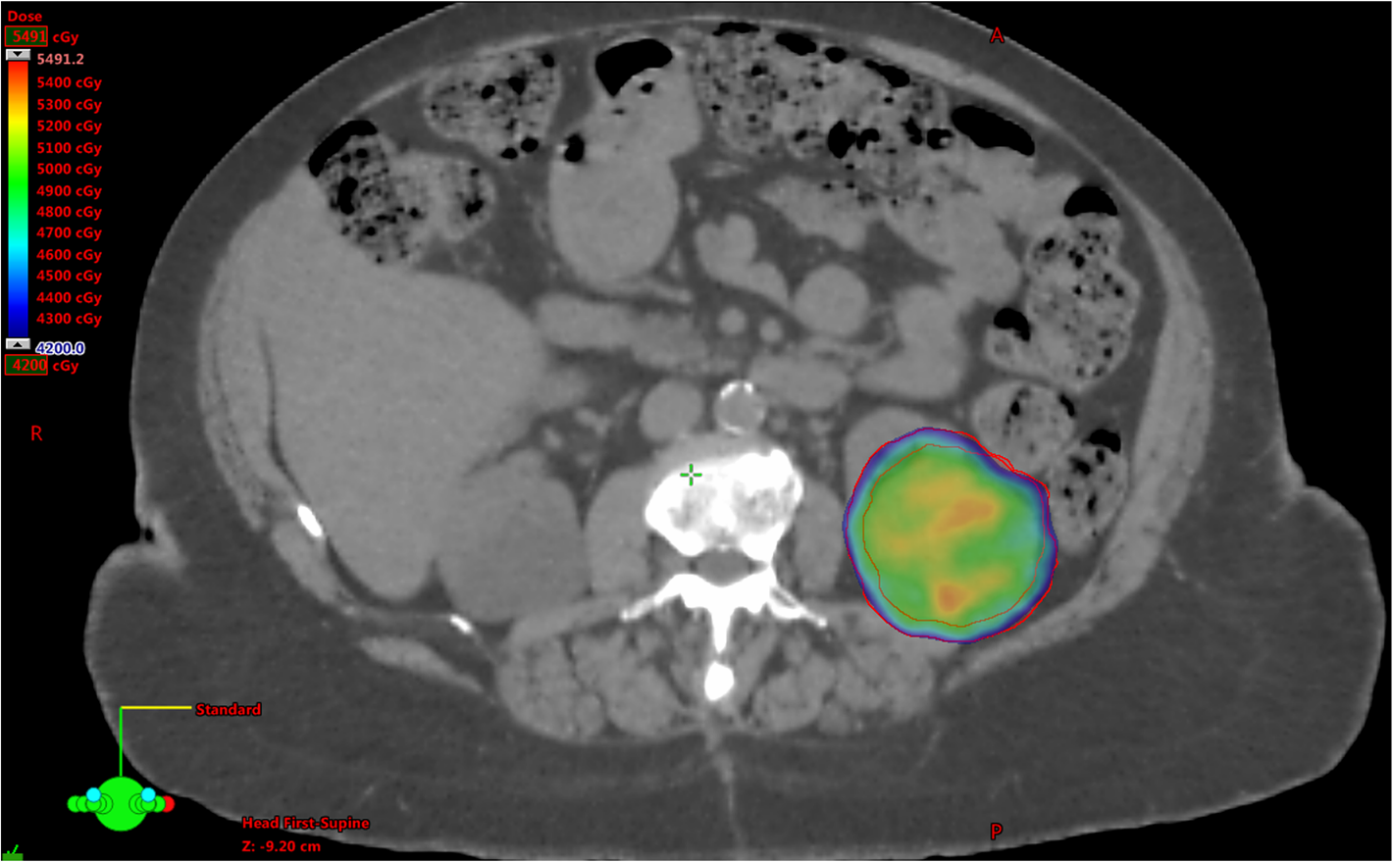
legend: Axial view of dosimetry as seen on planning CT demonstrates doses ranging from 42Gy. Doses greater than 42Gy are restricted to the planning target volume (outer contour), and the dose was reduced in areas that overlap bowel. Note that due to the haemorrhage, treatment was suspended after 14Gy had been delivered
